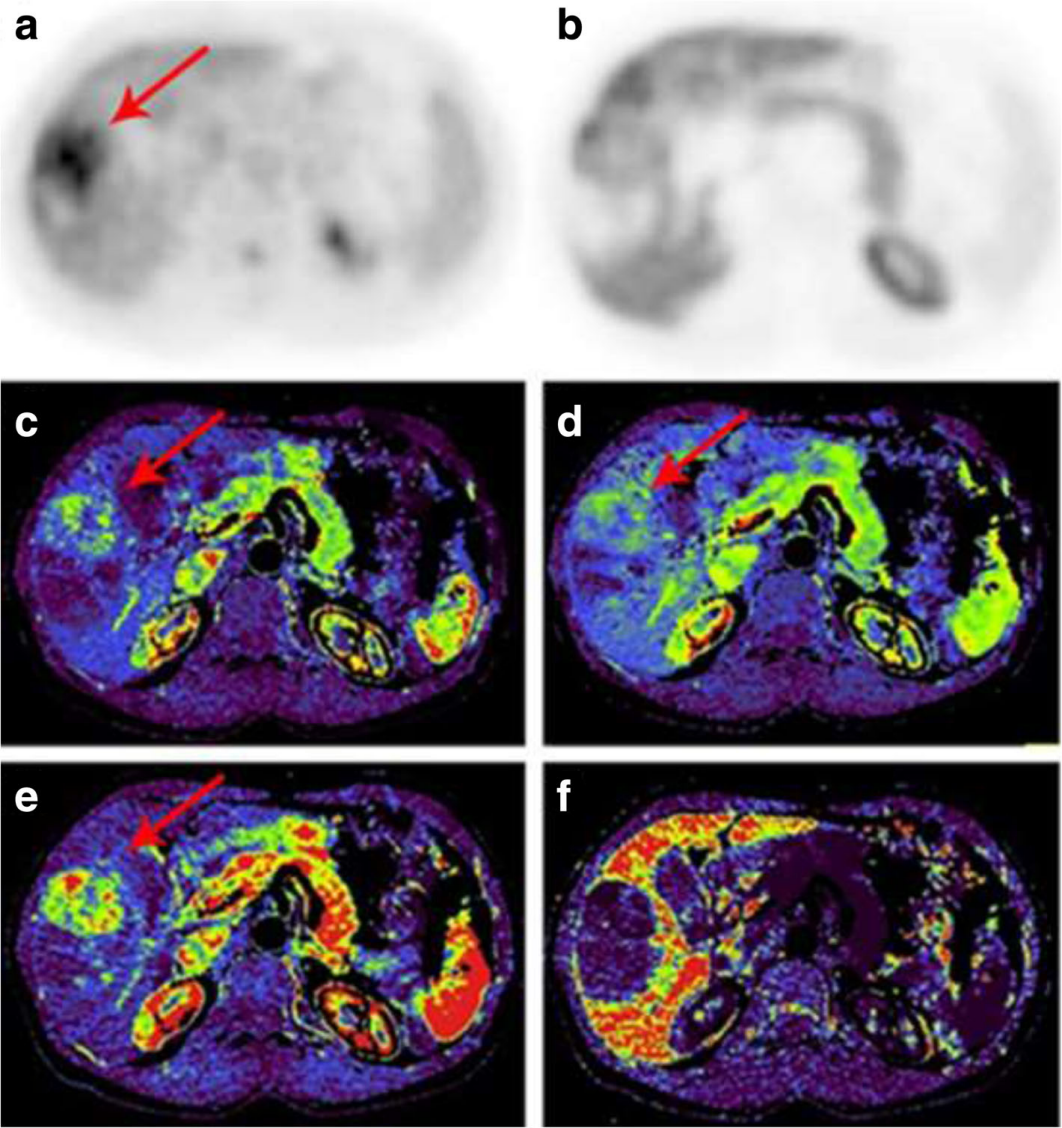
PET/CT and CT-perfusion in low-differentiated hepatocellular carcinoma. Patient B. Low-differentiated hepatocellular carcinoma. Images of axial slices of PET with 18F–FDG (a), 18F–FCh (b), BF perfusion maps (c), BV (d), ALP (e), PVP (f). Nodal formation in the right lobe of the liver (arrows) accumulates 18F–FDG (a), does not accumulate 18F–FCh (b). Perfusion maps visualize increased intensity and volume of arterial blood flow in the tumor (c, d, e), decrease of the portal component (f)
